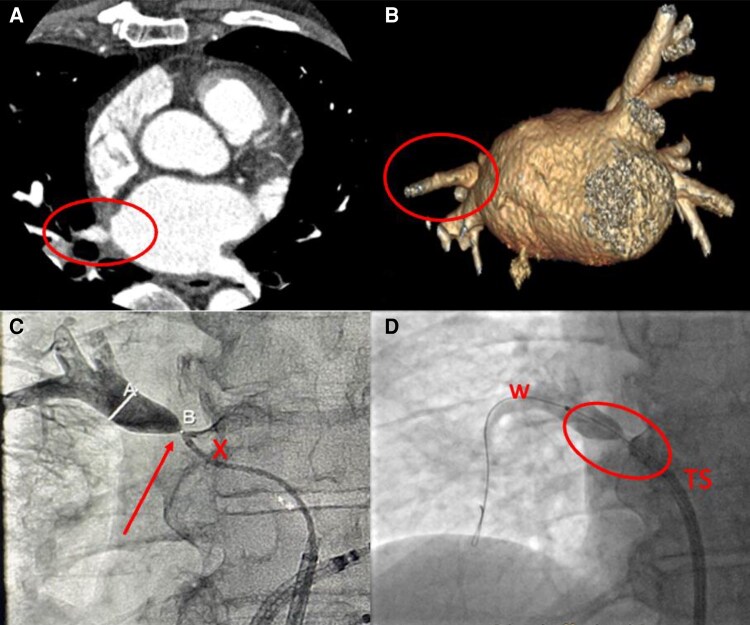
A patient with history of a prior PV isolation procedure and history of recurrent pneumonia in the right superior lobe following the procedure. *A*) CT showing high-degree stenosis of the right superior PV (circle), *B*) 3D reconstruction of the left atrium demonstrating a high-degree ostial stenosis of the right superior PV (circle), *C*) angiography of the right superior PV after transseptal access through a coronary diagnostic catheter (x) showing high-degree stenosis (arrow) (*A* indicates diameter of PV after stenosis, *B* site of stenosis), *D*) contrast injection into right superior PV after wiring (w) of stenosis (red circle) with transseptal sheath close to PV ostium. CT, computed tomography; PV, pulmonary vein.
